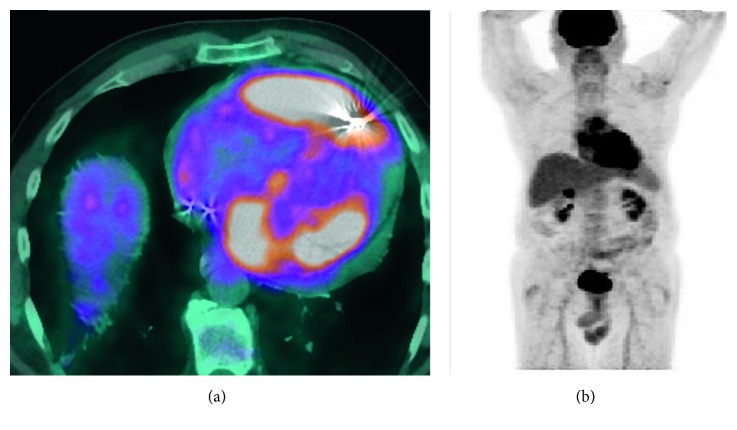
PET scan showing (a) increased FDG uptake in the cardiac tissue and (b) no extra cardiac FDG uptake.
